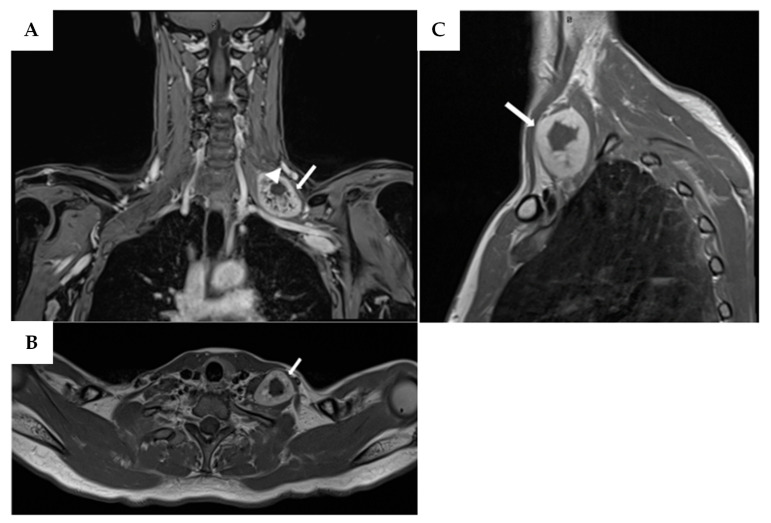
Enhanced T1-weighted-TSE MRI of patient 2 showing in the three axes the neoplasm at the base of the neck on the left brachial plexus. (A) Coronal view, the mass (white arrow) is located just above the subclavian artery and shows a central area of necrosis (arrowhead). (B) Axial view, showing the relationship of the mass with the scalene muscles. (C) The sagittal view reveals the close position in respect to the collar bone and the sternocleidomastoid muscle.
